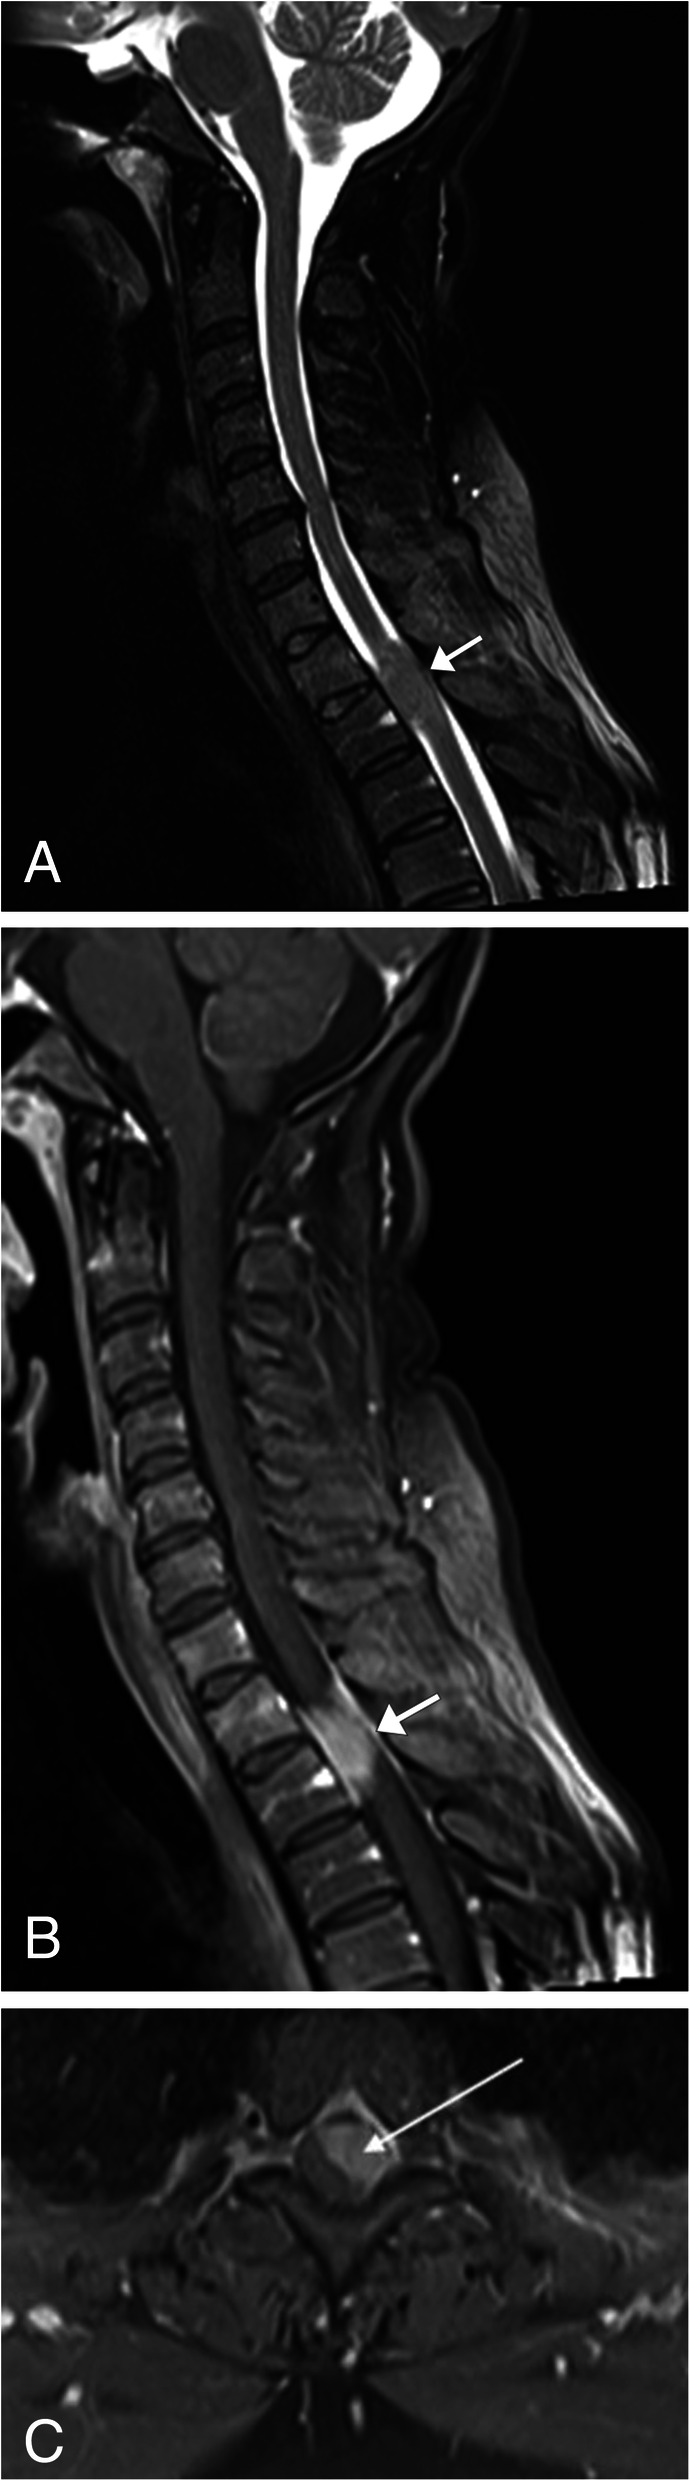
Meningioma. a Sagittal T2WI MR image of the cervical spine shows a well-circumscribed T2 hypointense lesion within the spinal cord at the level of T1–T2 (arrow). There is no evidence of increased T2 signal of the spinal cord to suggest edema. b Sagittal T1WI MR post contrast image and (c) axial TIWI post contrast of the cervical spine shows homogenous enhancement of the lesion. Note adjacent dural tail sign (short arrow). The axial image confirms the extramedullary location of the lesion (long arrow), as the cord is displaced to the right aspect of the spinal canal
